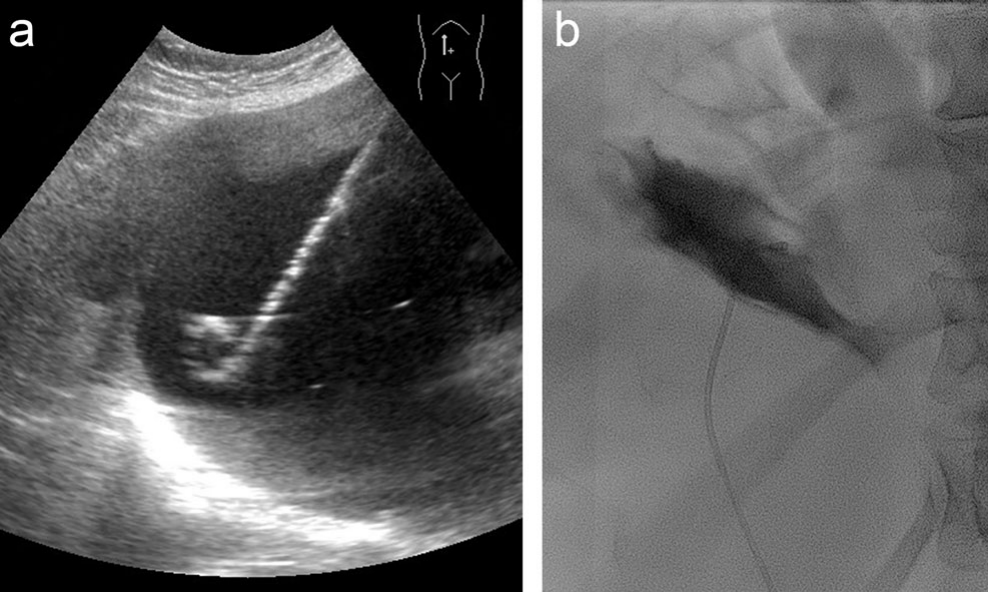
Ultrasonography-guided placement of pigtail catheter to perform aspiration (a) followed by fluoroscopy demonstrating a collapsed hepatic cyst without leakage (b)
